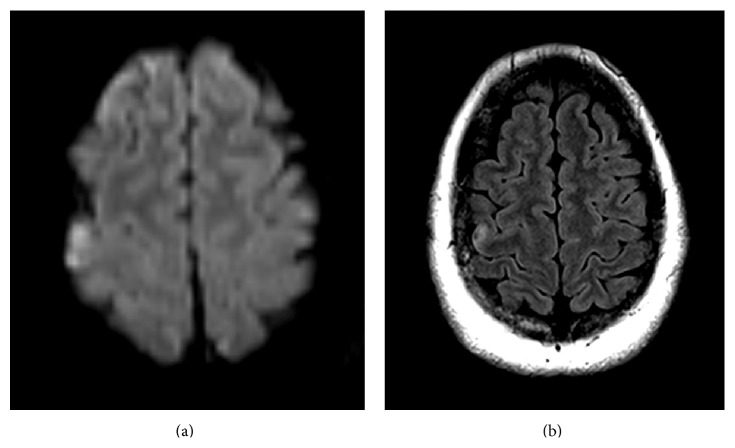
Restricted diffusion on DWI with increase T2 signal on FLAIR and postcontrast enhancement in right frontal lobe is consistent with subacute infarct within the right precentral gyrus.
